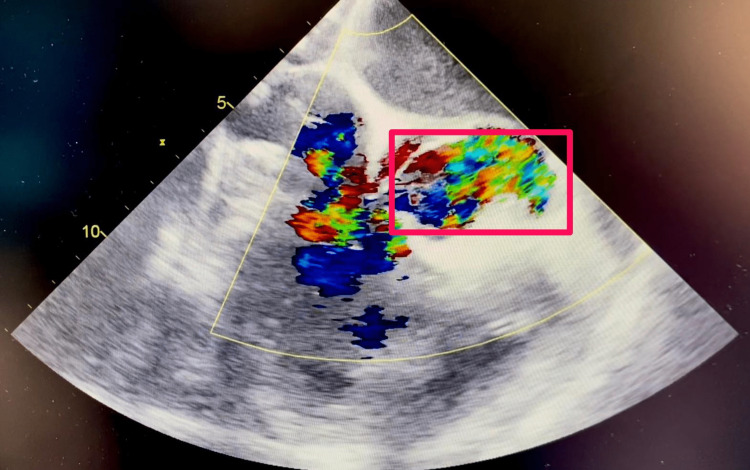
Color doppler transesophageal echocardiogram. Pink square shows an eccentric jet secondary to severe aortic insufficiency through the left coronary leaflet.
